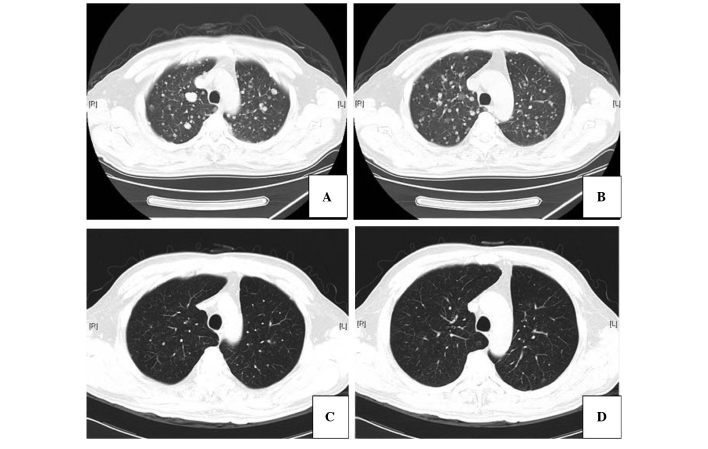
Axial chest computed tomography (CT) scans. (A and B) Scans prior to treatment with gefitinib. (C and D). Scans two months after treatment with gefitinib.
